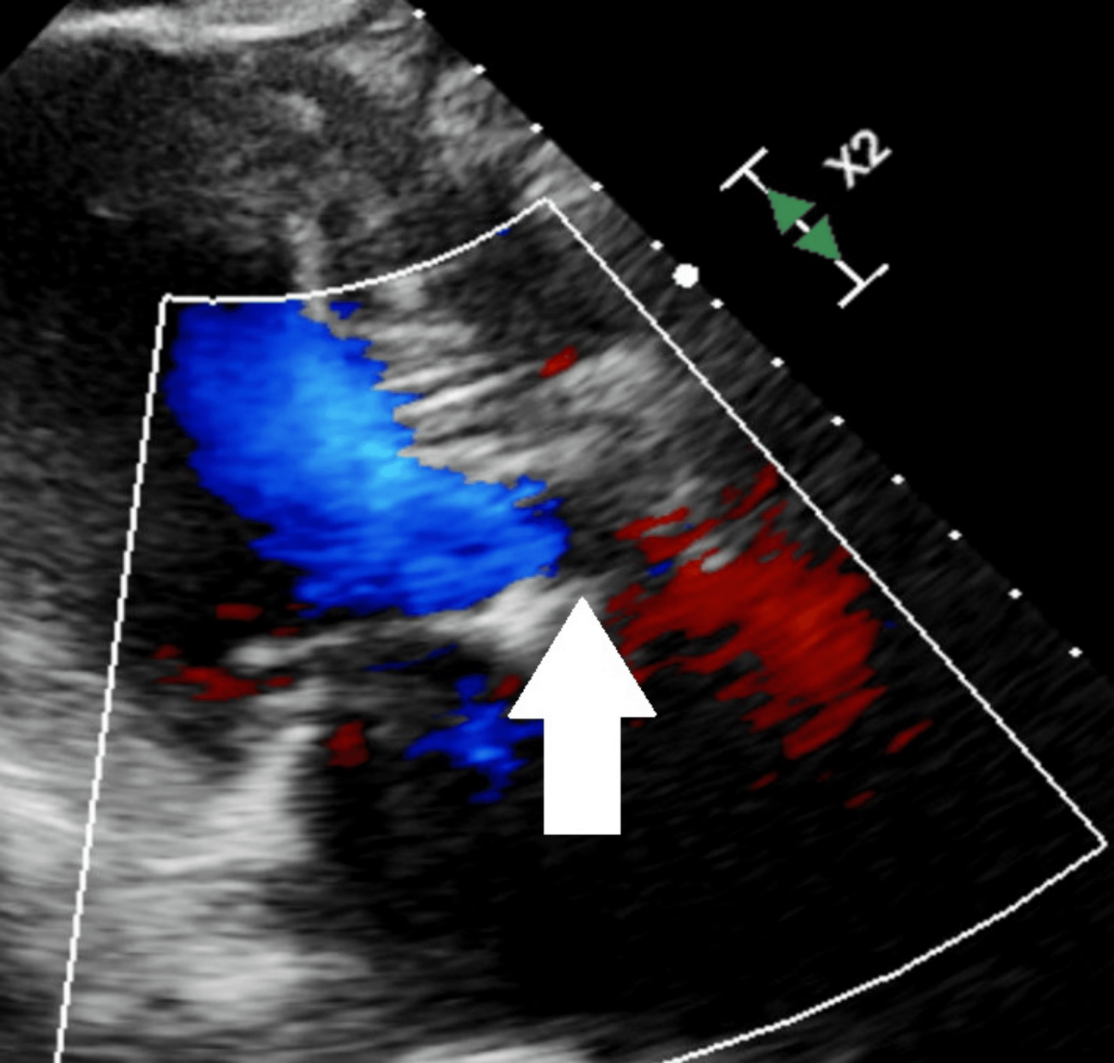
Post-procedure Transthoracic Echocardiogram (TTE) Post-procedural TTE demonstrating a well-positioned 26 mm SAPIEN Ultra Resilia valve (white arrow), with improved parameters: aortic valve gradient = 11 mmHg, LVOT/AV ratio = 0.53, velocity = 1.7 m/sec, LVEF = 65%, and transvalvular stroke volume index = 41 mL/m^2^. LVOT: left ventricular outflow tract; AV: aortic valve; LVEF: left ventricular ejection fraction
